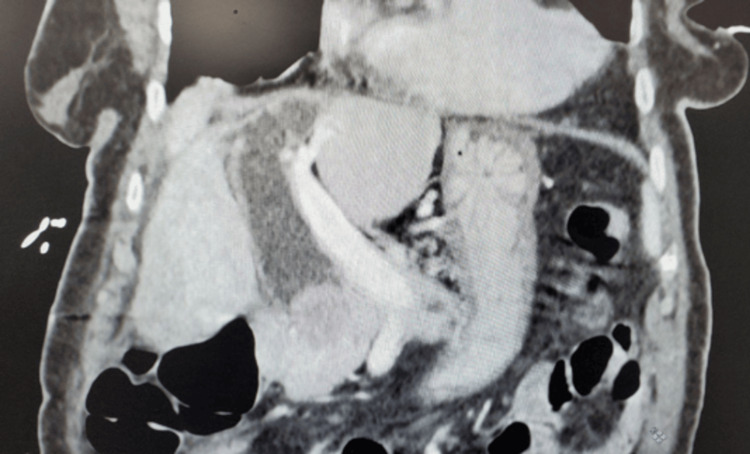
Coronal CT with contrast with large distal CBD stone measuring 24x27x33 mm. CT: computed tomography; CBD: common bile duct
